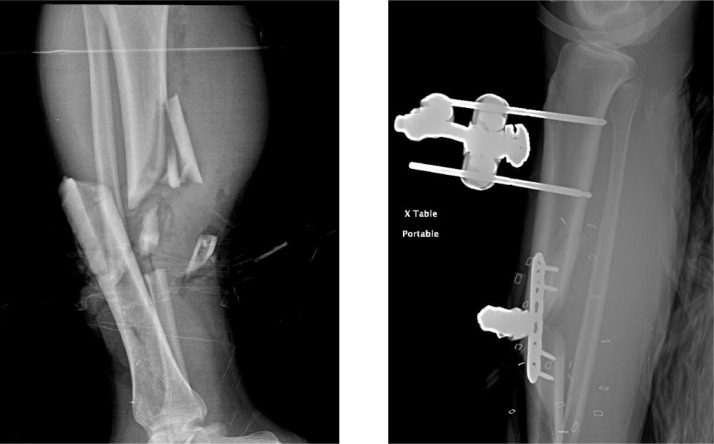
The radiograph of the right tibia-fibula fracture before (*left*) and after (*right*) the open reduction internal fixation (ORIF) and external fixation device application.
